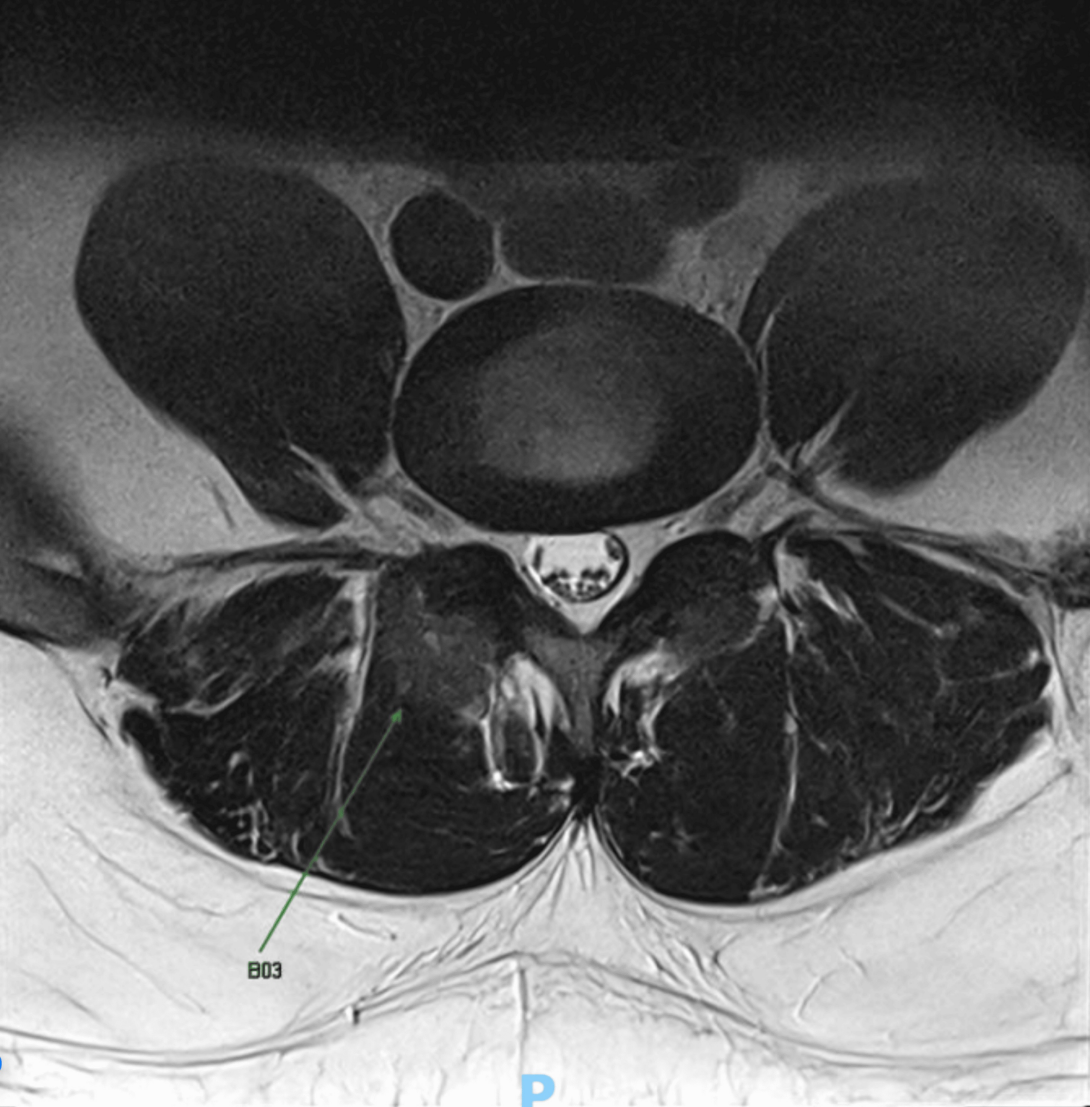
Contrast-Enhanced MRI Lumbar Spine Axial View, T2-Weighted
